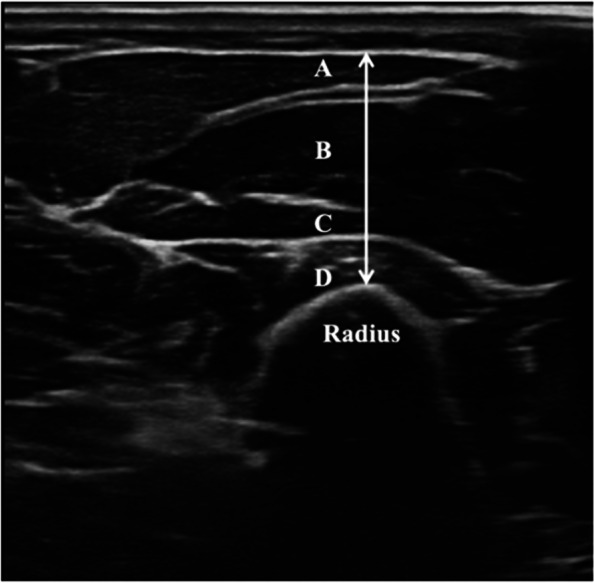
Cross-sectional sonogram of a healthy volunteer, Legend: Forearm muscles assessed: A, Brachioradialis muscle. B, Extensor carpi radialis longus muscle. C, Extensor carpi radialis brevis muscle. D, Supinator muscle
